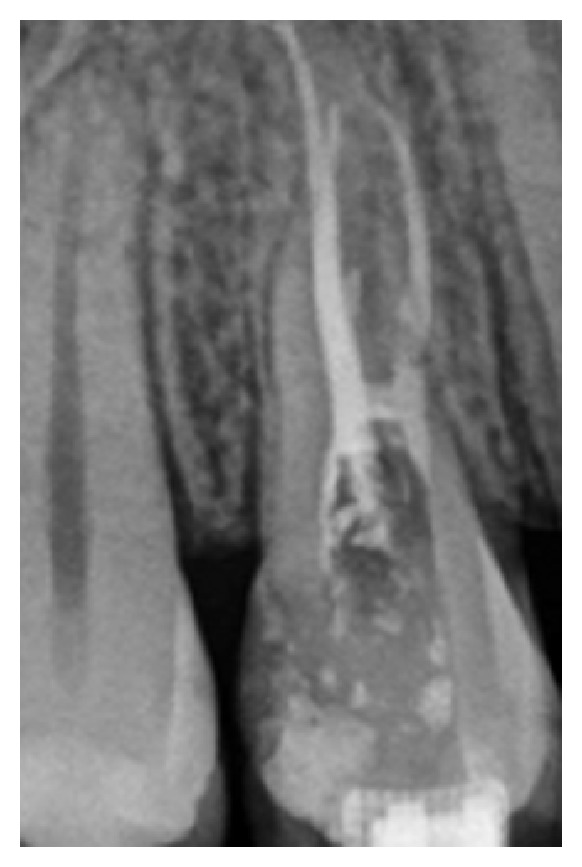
Final radiography after root canals filling.
